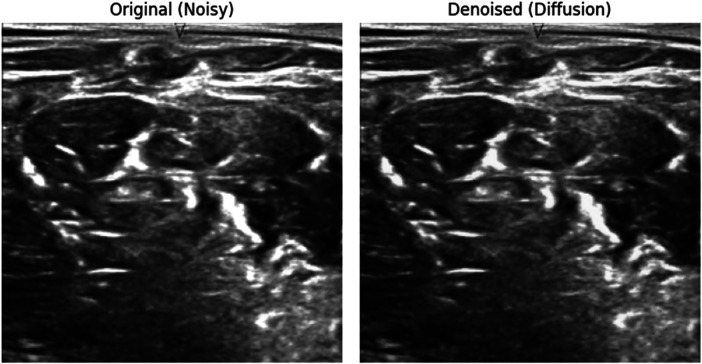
Qualitative visualization of diffusion-based denoising of ultrasound images, with the original noisy input image (left) and the denoised output image (right) obtained using the lightweight diffusion module.
